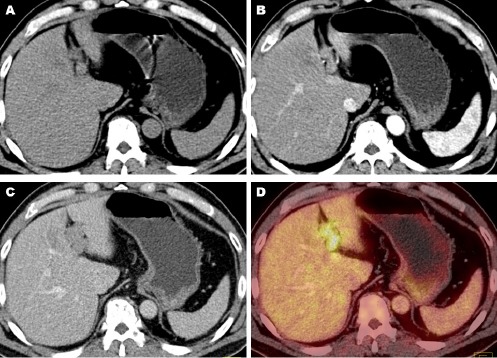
The contrast-enhanced FDG PET/CT shows the tumor thrombosis within the left expansile portal vein. The thrombus appeared isodense in the non-enhanced CT image (A), homogeneously contrast-enhanced in the arterial phase CT (B), and washed out in the portal venous phase CT (C). It showed an increased FDG uptake in the PET/CT image (D)
